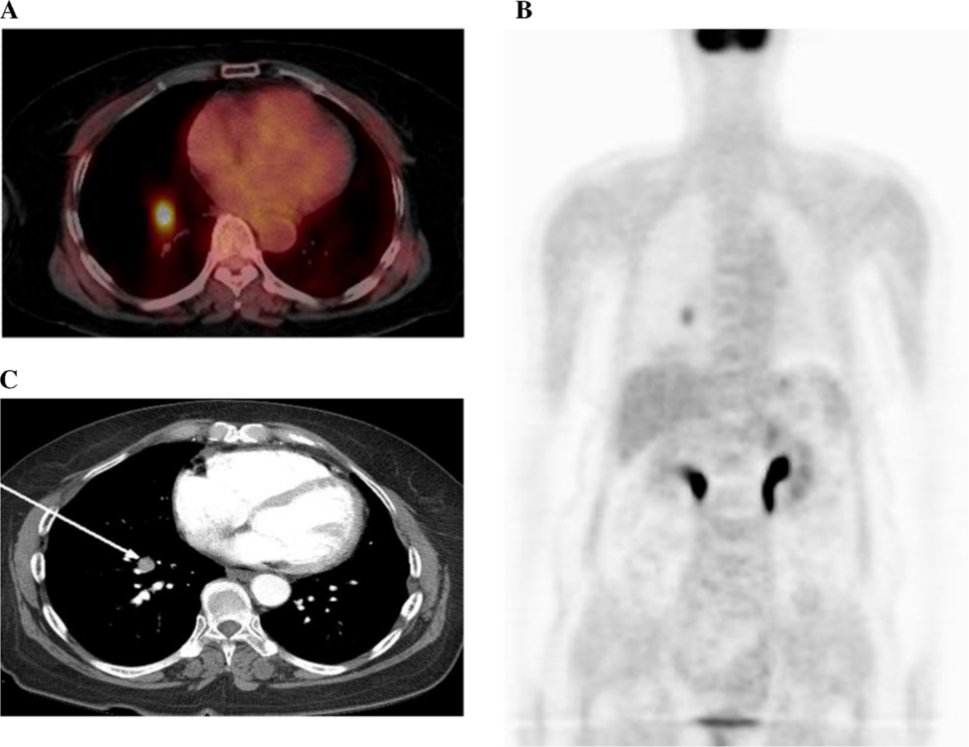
The F-18 fluorodeoxyglucose (FDG) positron emission tomography-computed tomography (CT) scan (A: axial view, B: coronal view) shows a focal increased FDG uptake lesion (maximum standardized uptake value, SUVmax = 6.5) (A, B). Chest CT scan shows an enhancing nodule measuring 1.3cm in the anterobasal segment of the right lower lobe (arrow) (C).
